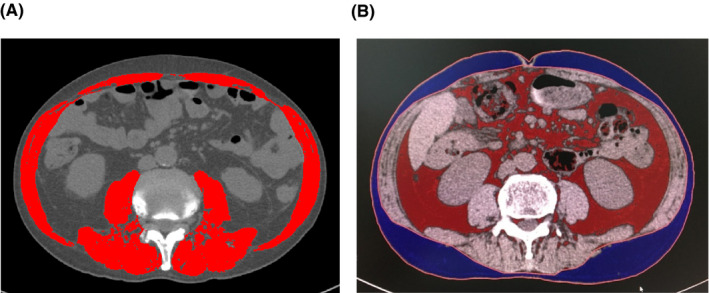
CT images for measurement of body composition. (A) The skeletal muscle area at the third lumbar vertebra level is highlighted in red. (B) The subcutaneous adipose tissue area is highlighted in blue and the visceral adipose tissue area is in red
